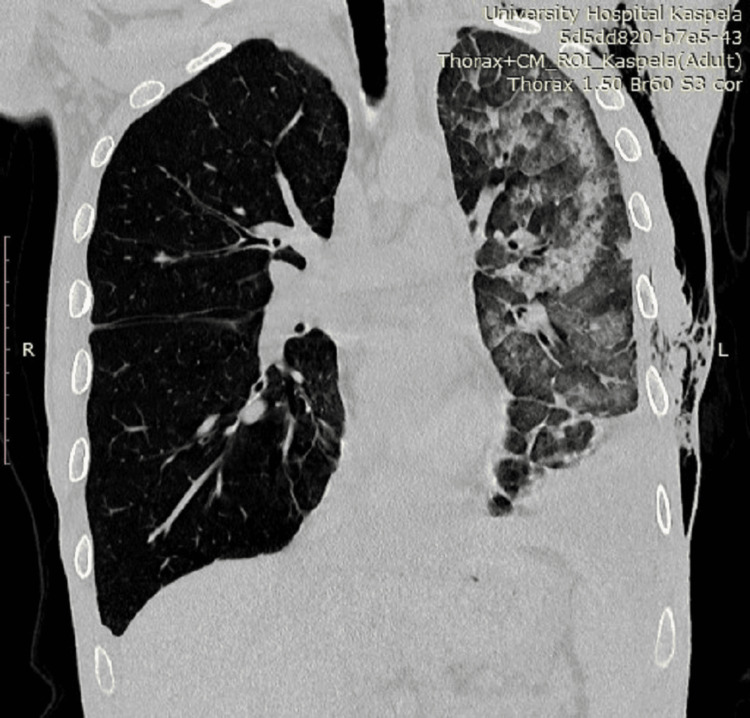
The infiltrative lesion in the parenchyma of the left lung, visualized on CT, as well as subcutaneous emphysema on the left side of the chest, a result of the carried out chest drainage
